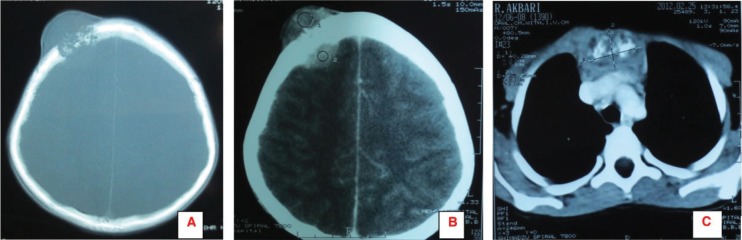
A, B: Brain CT scan shows an expansile bone lesion in the right frontal bone, invading the surrounding soft tissues. C: Chest CT scan shows a heterogeneous mass in the manubrium of the sternum composed of soft tissue and bone components.
